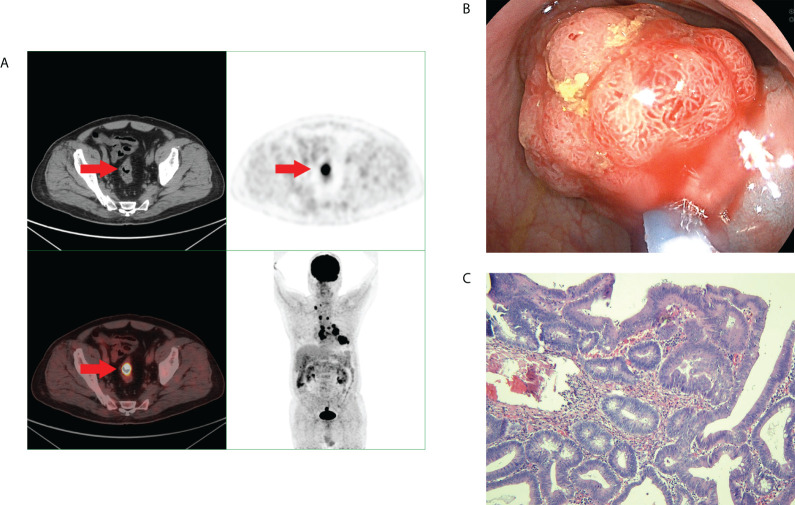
A middle-aged man underwent 18F-FDG PET/CT for left lung squamous cell carcinoma. Incidental focal 18F-FDG uptake was found in thesigmoid colon. The patient underwent colonoscopy and pathology examination. (A) Abnormal 18F-FDG PET/CT uptake in the sigmoid colon. (B) shows a sigmoid colonpolyp with a diameter of 2.5 cm. (C) shows the pathological pattern of villous tubular adenoma with high-grade intraepithelial neoplasia.
